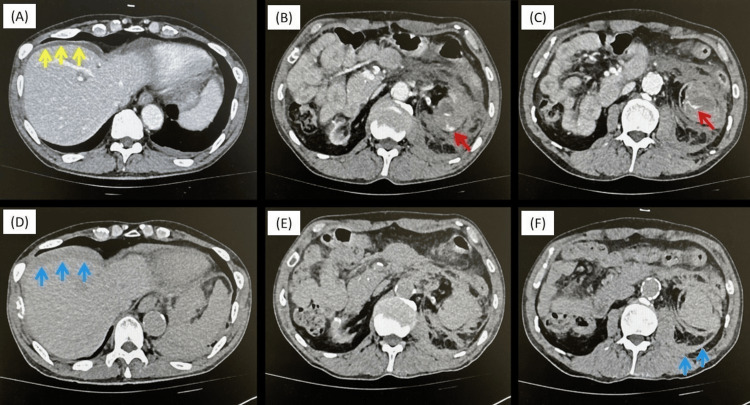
CT of the abdomen A-C, CT at the ER department. Extravasation from the lower branch of the renal artery (red arrow) and hematoma around the liver (yellow arrow) are observed. D-F, same slices with A-C of CT taken on the second day of hospitalization. An increase of hematoma around the liver and left lower pole of the kidney was observed (blue arrow).
